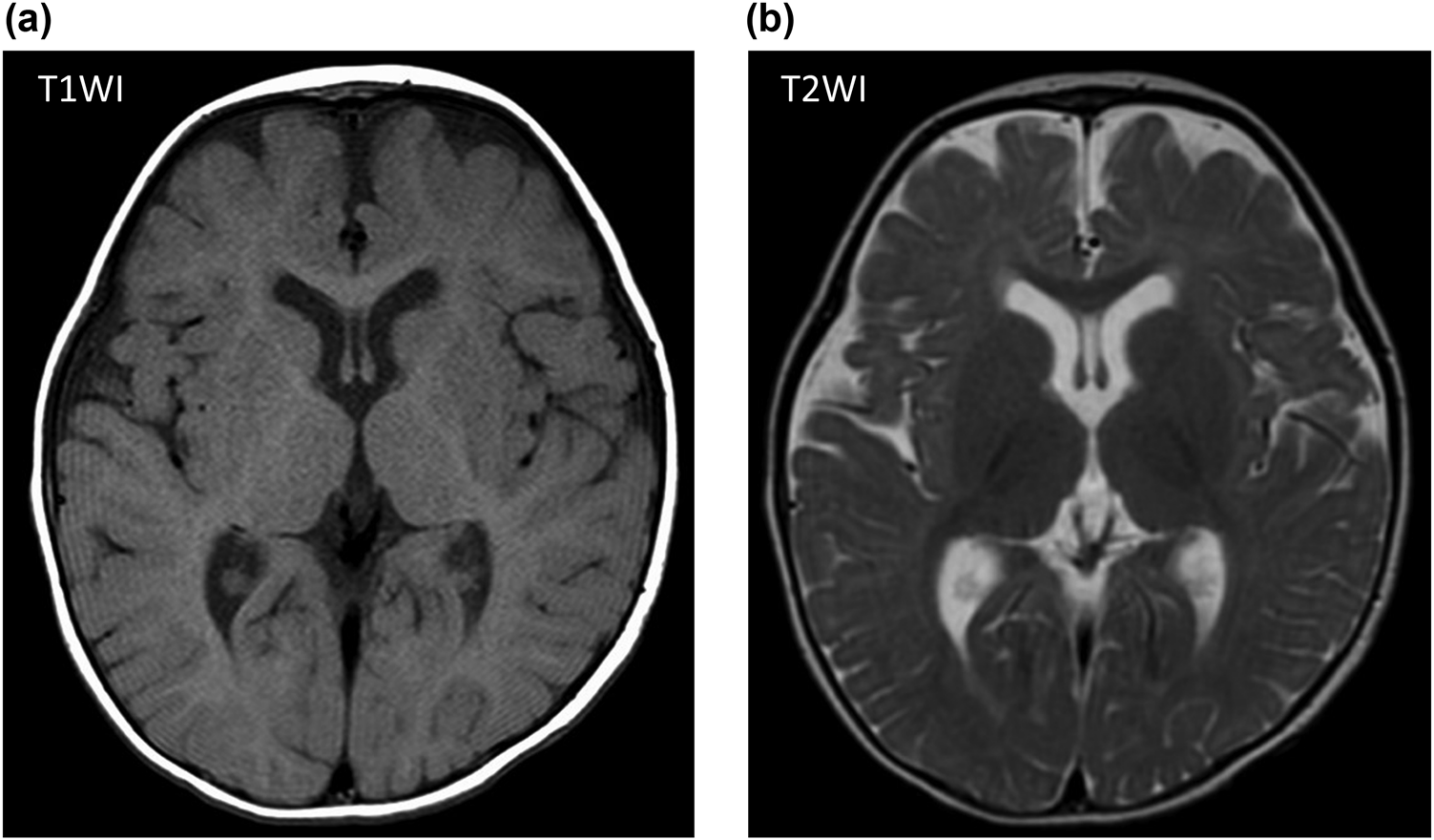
Brain magnetic resonance imaging scans of the patient at 1 year of age. (a) Axial T1-weighted imaging did not show hypointensities in the globus pallidus bilaterally (a sign of kernicterus) and (b) T2-weighted imaging did not show areas of increased signal intensity in the globus pallidus bilaterally (a sign of kernicterus).
